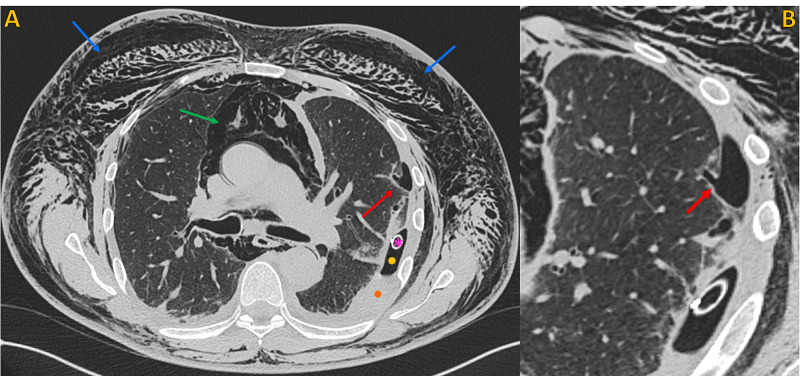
Non-contrast chest CT, lung window Non-contrast chest CT, lung window. A) Axial plane and B) Oblique axial plane (magnified view), demonstrates the point of communication between a peripheral enlarged bronchiolar structure and the pleural space, compatible with a bronchopleural fistula (red arrow). Note extensive soft tissue emphysema (blue arrows), large pneumomediastinum (green arrow), left pneumothorax (yellow circle), and left pleural effusion (orange circle). Chest tube (pink asterisk).
